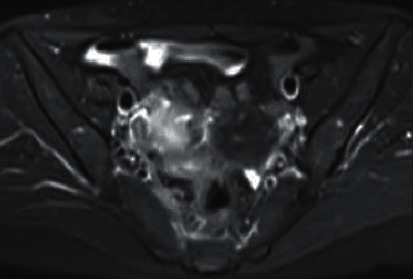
Original MRI image of an FPA patient.
